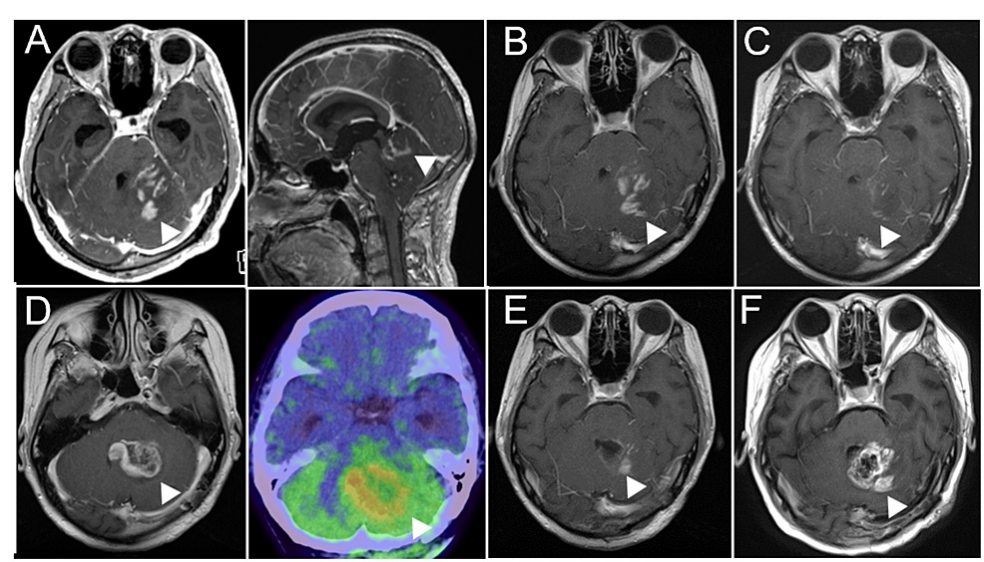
MRI and PET images during the clinical course of Case 1. (A) MRI contrast-enhanced T1-weighted axial (left) and sagittal (right) images at initial onset. (B) MRI image after chemotherapy with cisplatin and vincristine. (C) MRI image after radiation therapy (54Gy/30fr). (D) MRI image (left), and Methionine PET (right) at one month after radiation therapy. (E) MRI image after tumor removal. (F) MRI image after three months of tumor removal.
